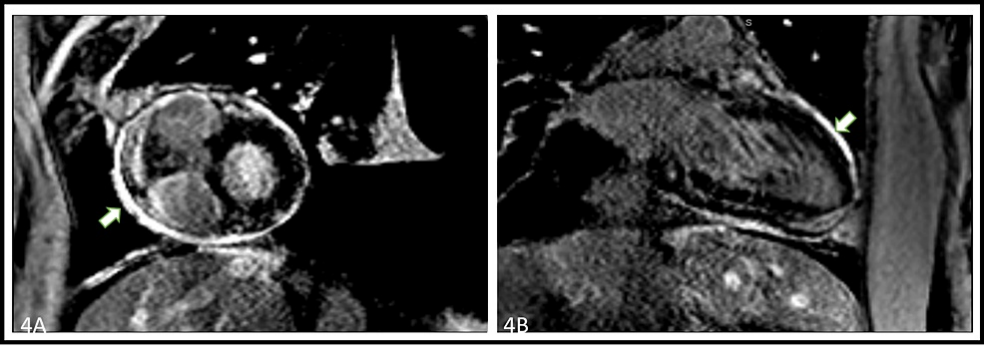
Cardiovascular magnetic resonance imaging mono-images demonstrating late gadolinium pericardial enhancement suggestive of active pericardial inflammation
